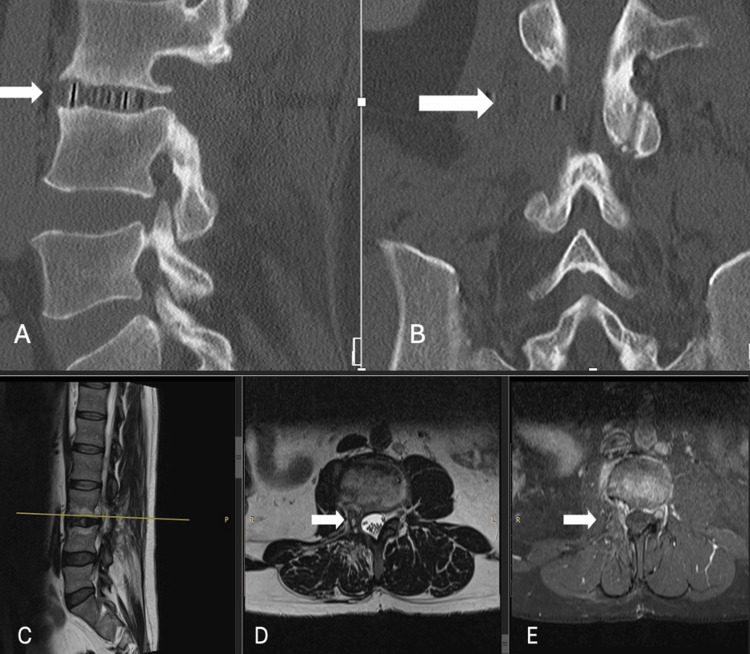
CT scans (sagittal (A) and coronal (B) views); MRI (sagittal (C),axial T2 (D), and axial T1 Gd (E) Post-surgical changes with L3-L4 intersomatic cage (lateral lumbar interbody fusion (LLIF)) and resection of the right L3-L4 facet joint (arrow in A and B). The MRI shows gadolinium (Gd)-enhancing scar tissue in the right perivertebral location, in the ipsilateral foraminal and paraspinous canals (arrows in D and E).
